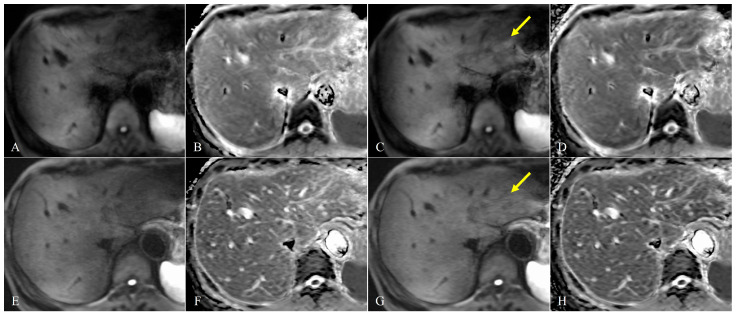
(A) BH non-ACSA (conventional) DWI and (B) ADC map, (C) BH-ACSA DWI and (D) ADC map, (E) FB non-ACSA (conventional) DWI and (F) ADC map, and (G) FB-ACSA DWI and (H) ADC map. In both FB and BH imaging, the signal intensity of the liver parenchyma in the left lateral segment on ACSA DWI showed a distinct signal increase (arrow in (C,G)) compared to non-ACSA DWI. Overestimated ADC values of the lateral segment on the non-ACSA ADC map are improved on the ACSA ADC map in both FB and BH imaging. In the comparison between BH-ACSA DWI and FB-ACSA DWI, the SIR between the right hepatic lobe and left lateral segment was smaller on FB-ACSA DWI (1.74) than on BH-ACSA DWI (3.28) (arrow in (C,G)).
